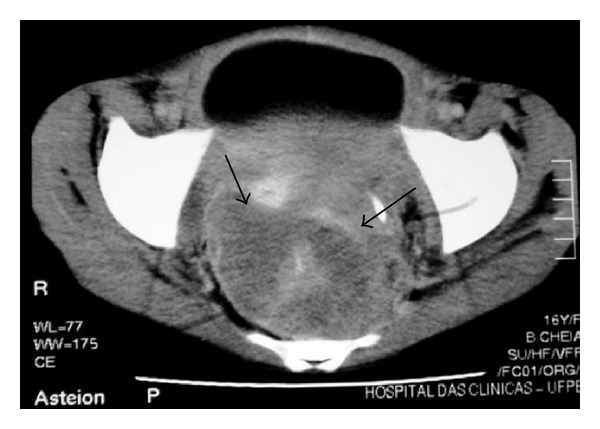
NHL in a 16-year-old girl. Axial contrast-enhanced CT of the pelvis reveals soft-tissue mass involving the rectal region (arrows).
